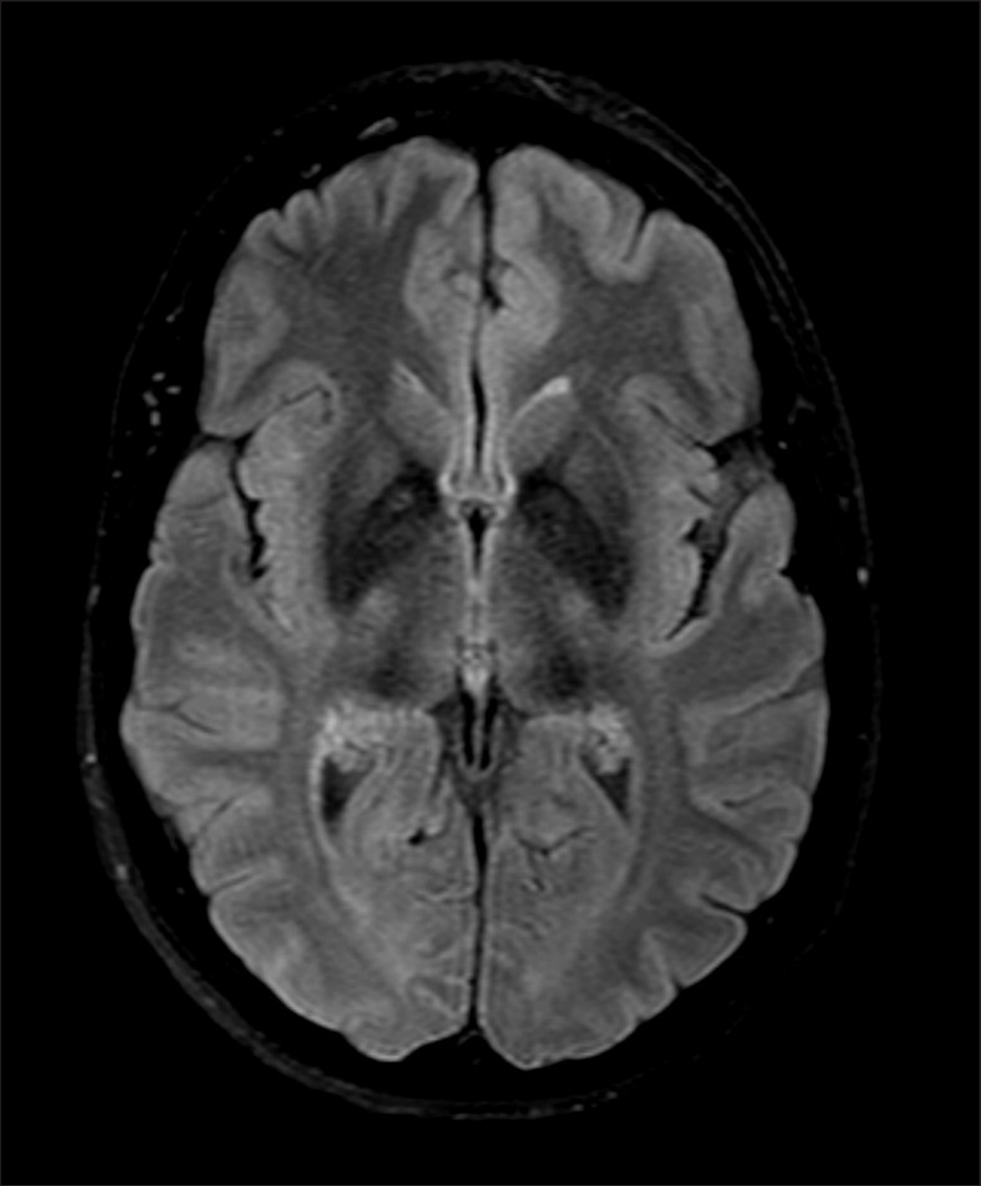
Brain MRI 2 years after symptom onset.
